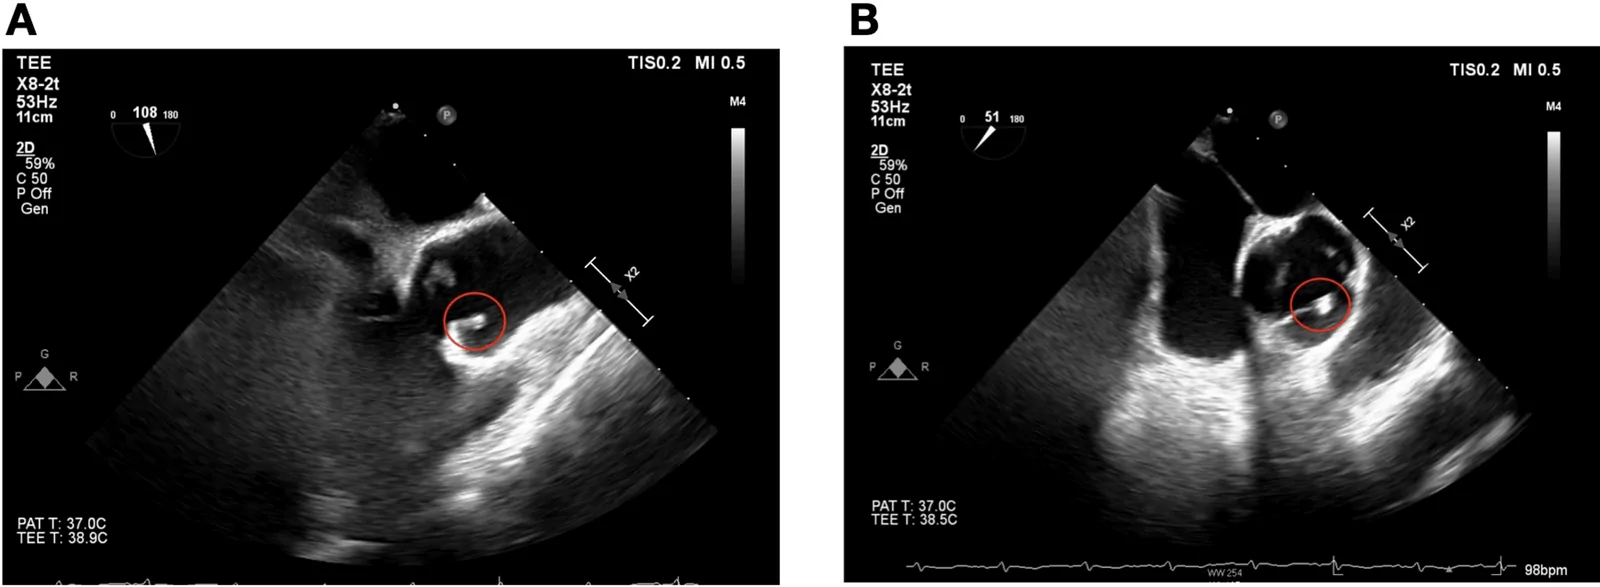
Transesophageal echocardiogram showing aortic valve vegetation TEE: transesophageal echocardiogram; NBTE: nonbacterial thrombotic endocarditis; ME AV LAX: mid-esophageal aortic valve long-axis; ME AV SAX: mid-esophageal aortic valve short-axis TEE demonstrating NBTE. (A) ME AV LAX view and (B) ME AV SAX view visualize a small, discrete lesion present on the aortic side of the right coronary cusp consistent with a sterile platelet-fibrin vegetation
